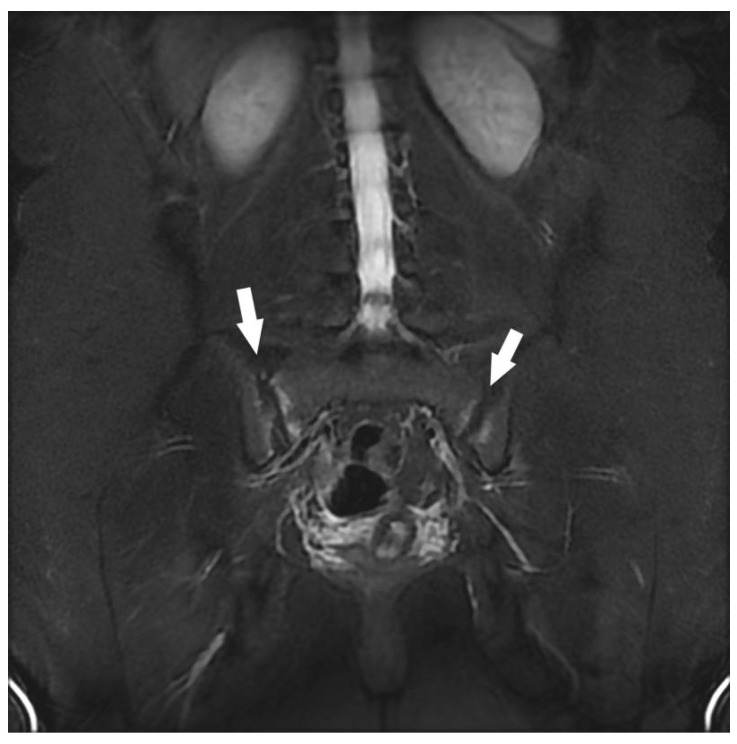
Assessment of right sciatica in a 27-year-old woman. Coronal STIR MRI. Bilateral hypersignal of subchondral bone (white arrows) involving the sacro-iliac joints (predominant on the right) with bone erosions of the right iliac edge.
